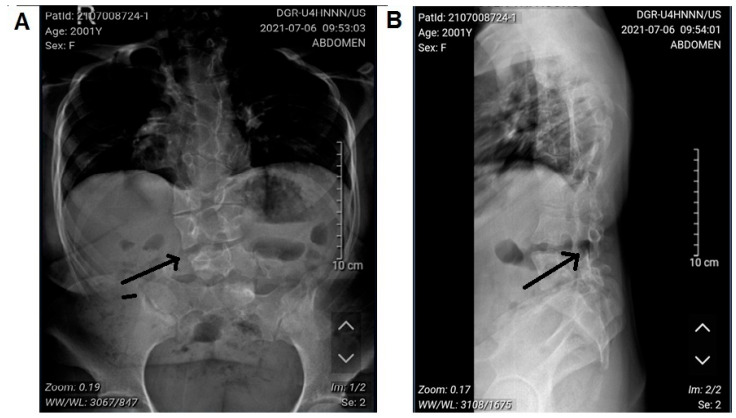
Abnormal X-ray images of the patient. (A). The patient’s X-ray shows a short chest with butterfly vertebrae and consolidation. (B). X-ray shows that the ribs are distorted and curved slightly. Black arrow points to the location of the deformed vertebrae.
